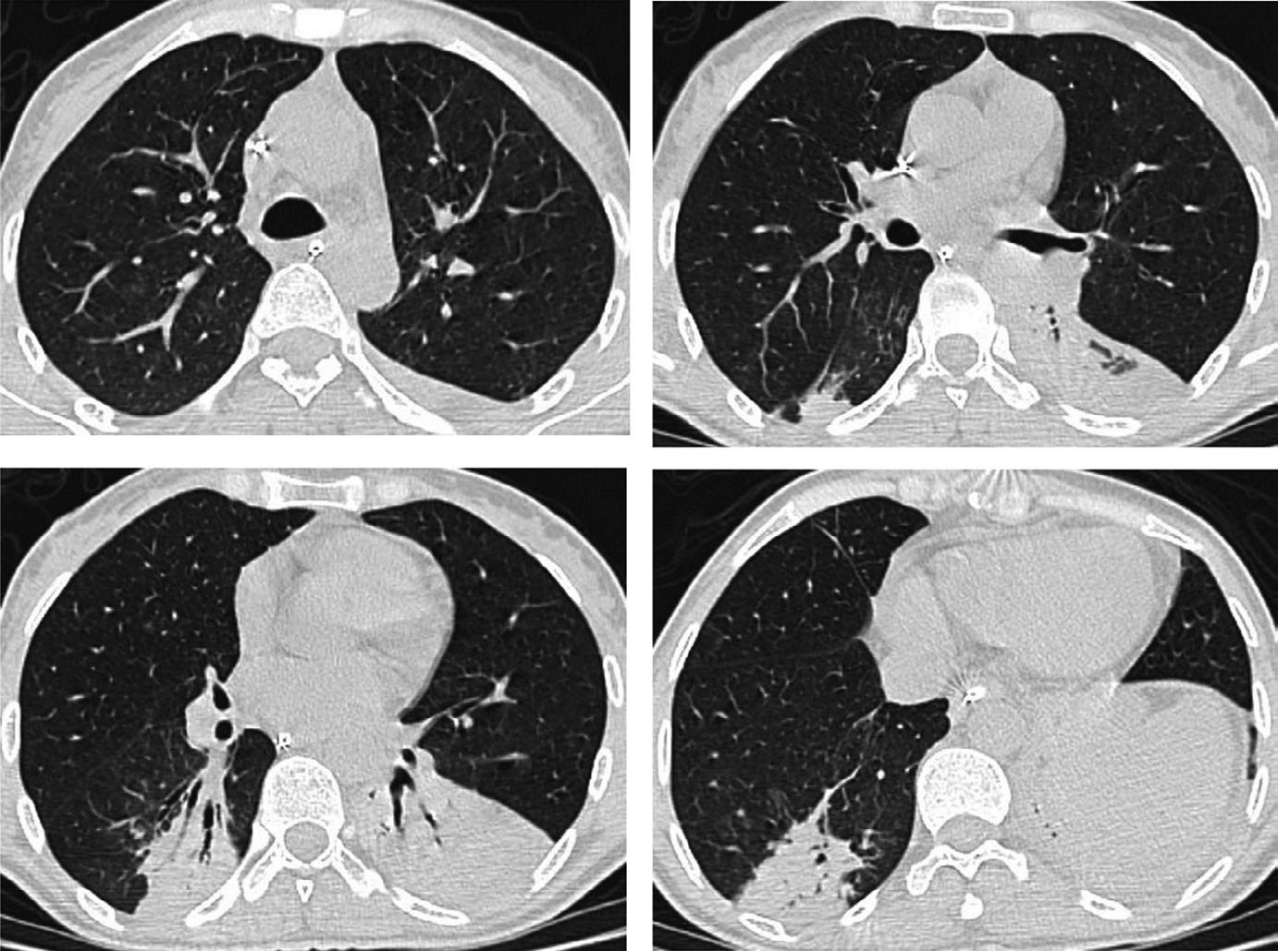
The control chest CT scan: Axial CT images have demonstrated the disappearance of previously described lesions in the upper lobes and middle lobe. It showed also a partially ventilated collapse of both lower lobes
